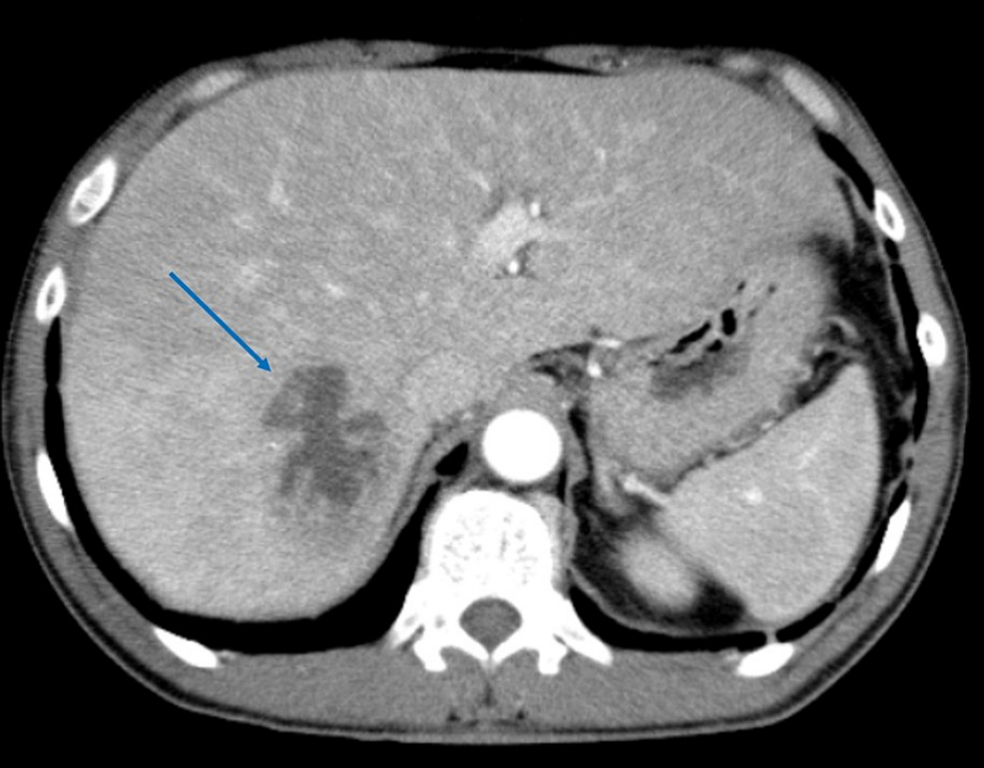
Computed tomography (CT) abdomen and pelvis with contrast The blue arrow indicates a septated hypodense mass in the right liver lobe, most likely representative of an abscess.
